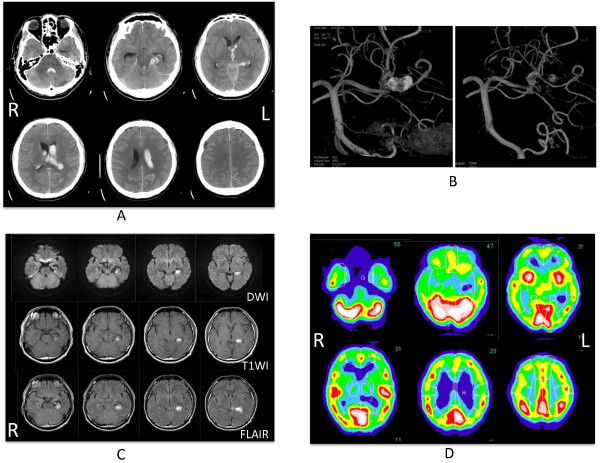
Neuroradiological examination. (A) A hematoma was observed in the left hippocampus, extending to the parahippocampal gyrus in a brain CT scan on admission. (B) Cerebral angiography before (left) and after (right) coil embolization. An irregular dissecting aneurysm was found in the distal portion of the left posterior cerebral artery. Dissection had occurred in the main trunk of the posterior cerebral artery, and it was formed due to a portion that extended saccular and a portion that extended in fusiform, where the portion that extended in saccular was embolized, and the main trunk was preserved. (C) A hematoma was found in the left hippocampus extending to the parahippocampal gyrus in MRI scan on day 10 after the onset. (D) A decline in cerebral blood flow in the left medial temporal lobe was found in SPECT images on day 14 after the onset.
